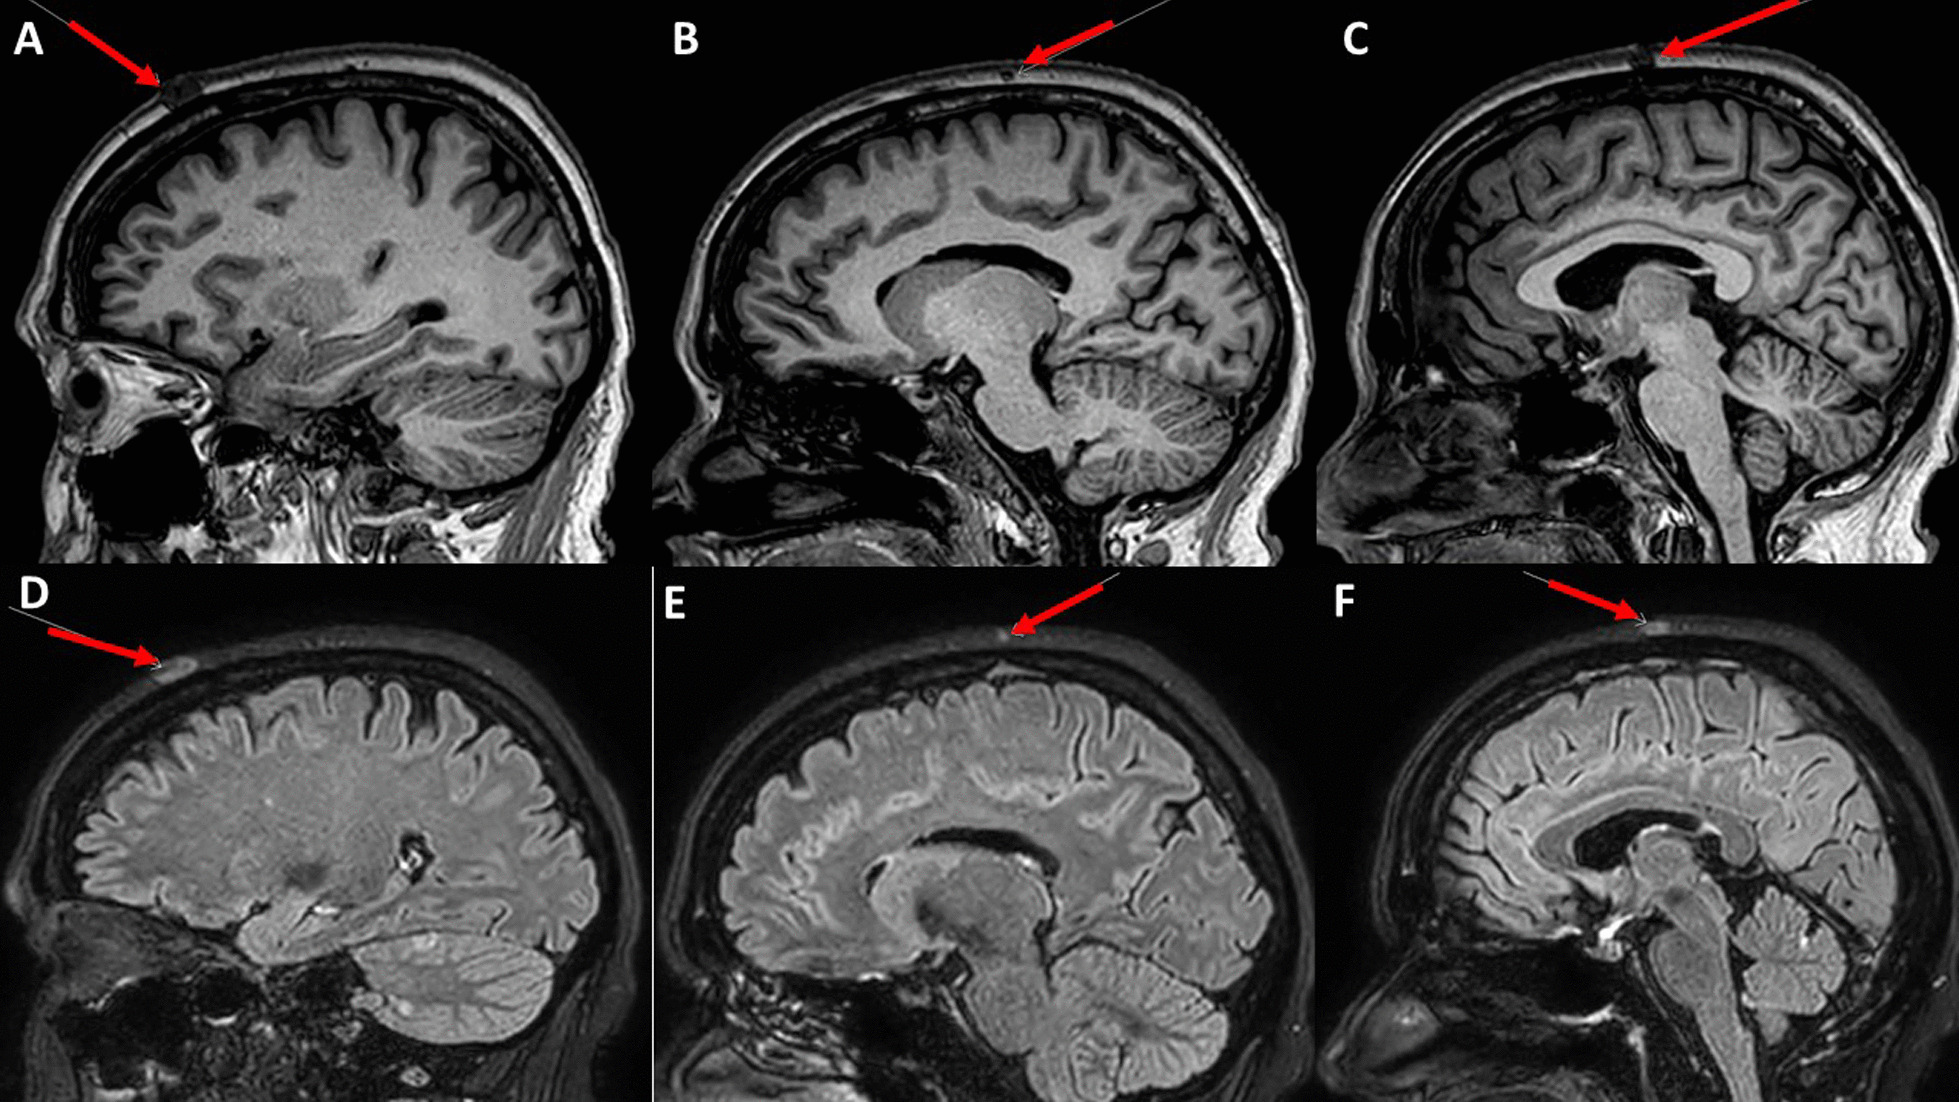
Selected sagittal images from brain MRI of T1 non-contrast and fluid-attenuated inversion recovery (FLAIR) post-contrast sequences showing three scalp lesions. A–C showed hypointense lesions on T1 indicated by red arrows. D–F showed hyperintense lesions on FLAIR. Lesions are indicated by red arrows. A & D showing the largest one on the left frontal region measures about 1.4 cm, 1cm, 0.3 cm at the time of examination.
